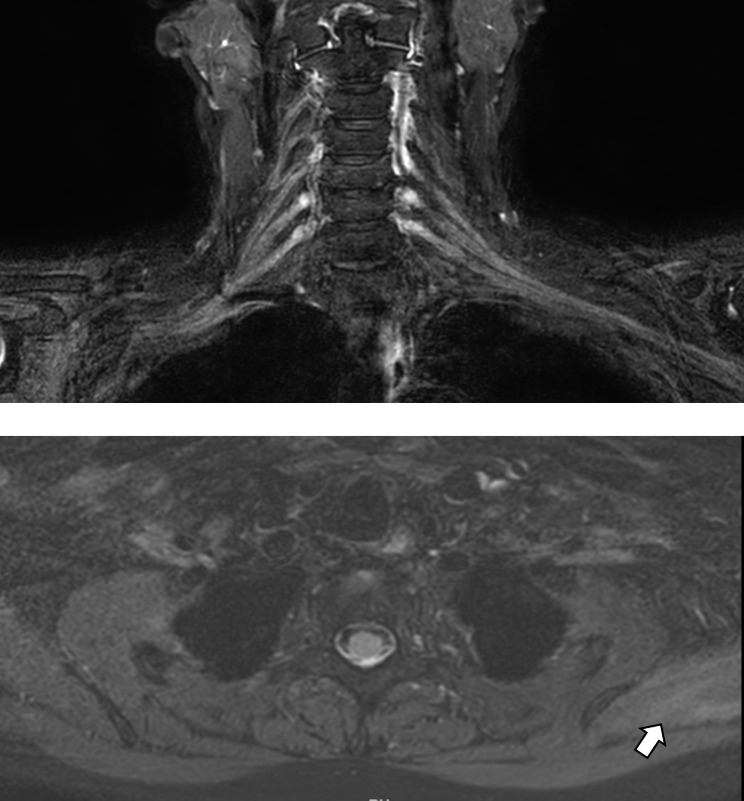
The images of magnetic resonance neurography (upper panel) and muscle magnetic resonance image (lower panel). The brachial plexus shows no significant abnormality. The white arrow indicates slightly increased T2 signal in the left supraspinatus muscle
